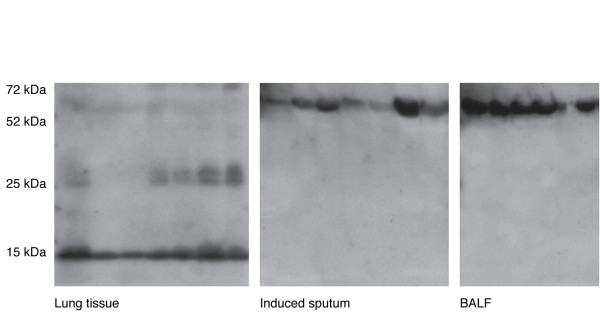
The expression of Hb by standard Western blot analysis in the lung homogenates (n = 6), BALF (n = 6) and induced sputum supernatants (n = 7) of control subjects. BALF had been obtained from subjects with prolonged cough who had normal spirometry, BAL cell profile and HRCT finding. Induced sputum was obtained from healthy non-smokers. The results indicate that the major Hb forms detectable by the commercial antibodies differ between the lung tissue and BALF or sputum, the major band in the lung tissue being the Hbα and Hbβ (not shown in this figure) complex, while it is detected as larger complexes corresponding to Hb tetramers in the "secretions". The expression by using the Hbα and β antibodies in the BALF and sputum was very similar further suggesting that the band represents tetramer. Here only Hbα is shown. For patient characteristics see Table 4.
